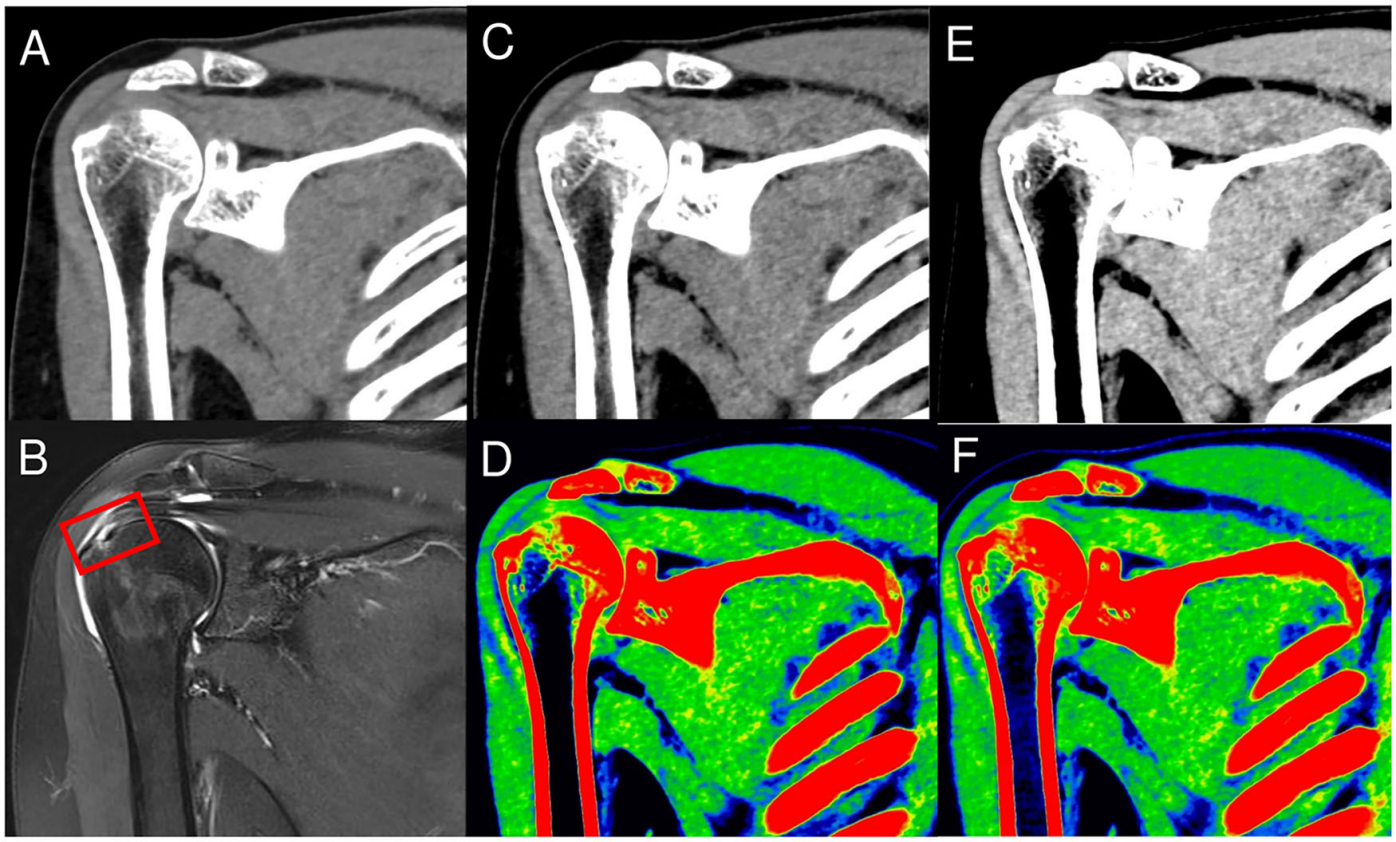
Oblique coronal plane images of a 52-year-old man with right supraspinatus tendon tear: (A) SCT image, (B) proton density weighted fat-saturated MRI image showing a tear in the supraspinatus tendon (red dotted rectangle), (C) DECT images with mono+ 50 keV, (D) color-coded DECT images with mono+ 50 keV, (E) DECT images with mono+ 90 keV, and (F) color-coded DECT images with mono+ 90 keV. SCT, standard computed tomography; DECT, dual-energy computed tomography; MRI, magnetic resonance imaging
